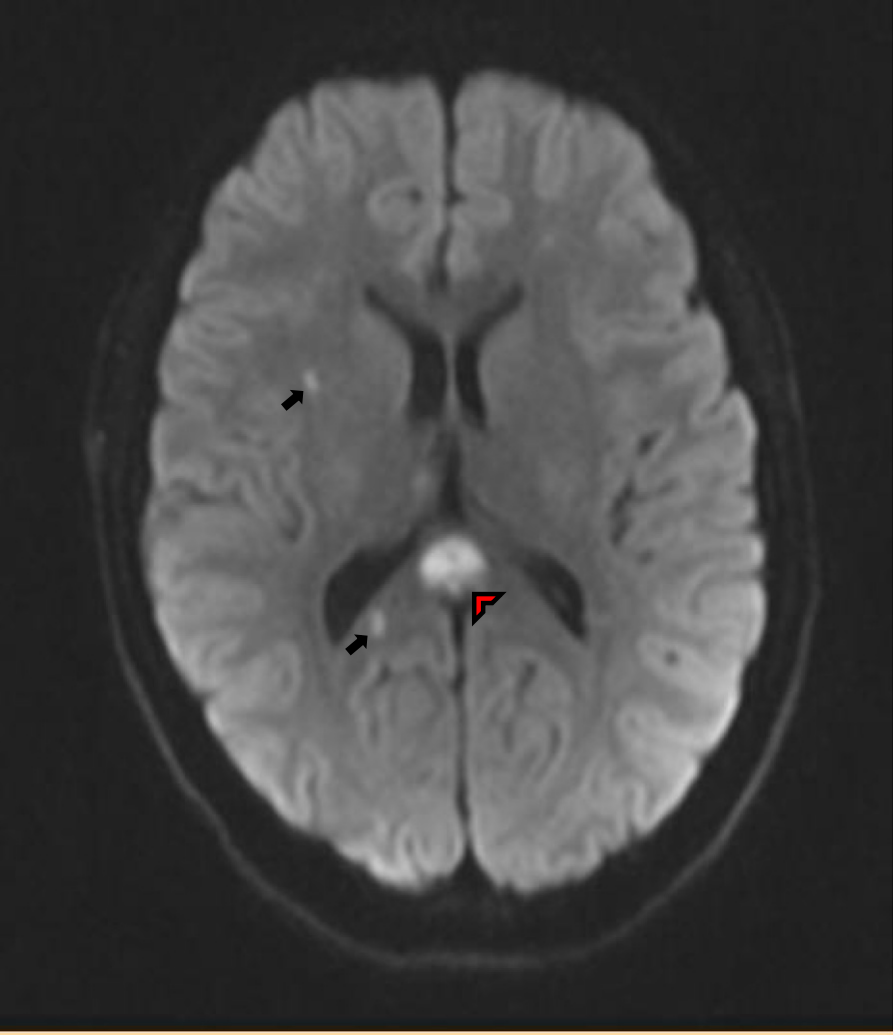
Axial brain magnetic resonance imaging sequences with diffusion-weighted imaging (DWI) revealed multifocal subacute punctate supratentorial white matter lesions (*black arrows*) with the most prominent lesion affecting the posterior corpus callosum (*red arrowhead*). The study also showed T2/fluid attenuated inversion recovery (FLAIR) hyperintensities involving the deep gray nuclei as well as leptomeningeal enhancement (not shown)
